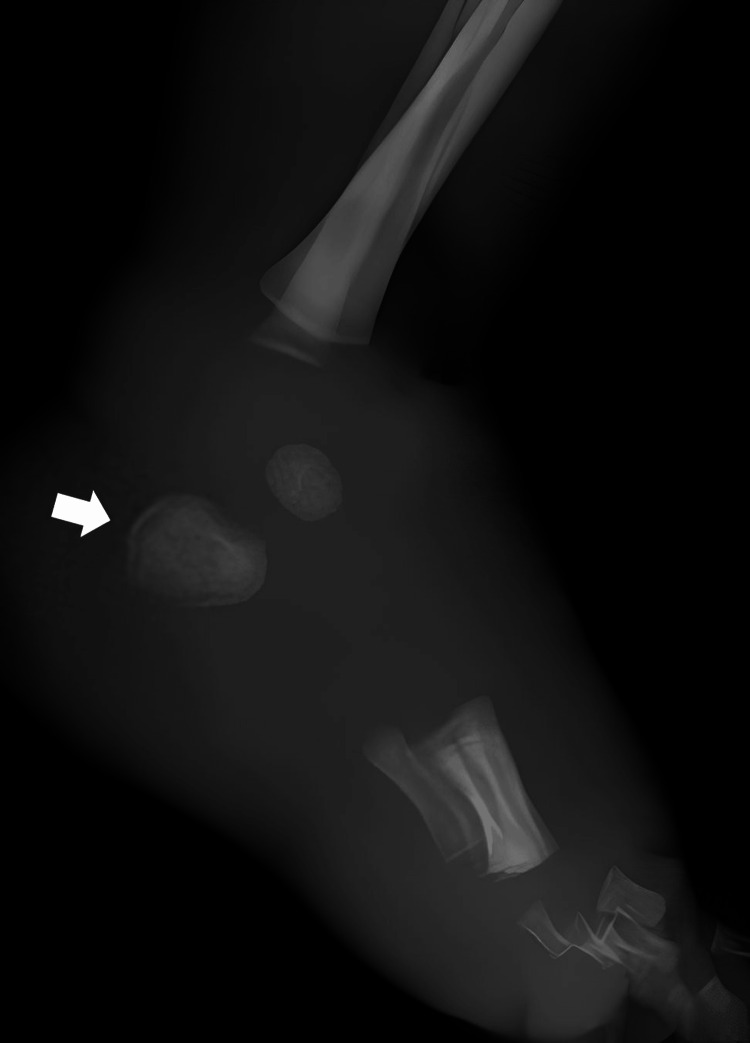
Radiograph showing osteolysis at the base of the calcaneus. The arrow points to the calcaneus.
